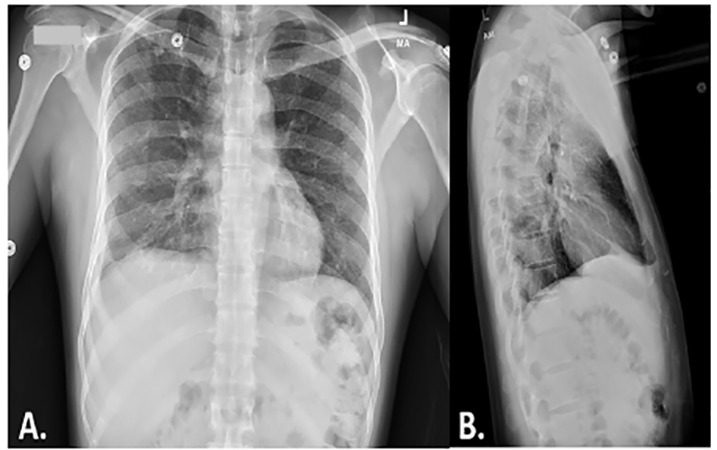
Initial chest radiograph with reticulonodular diffuse lung pattern that can be seen in infection. A) anteroposterior view. B) lateral view.
